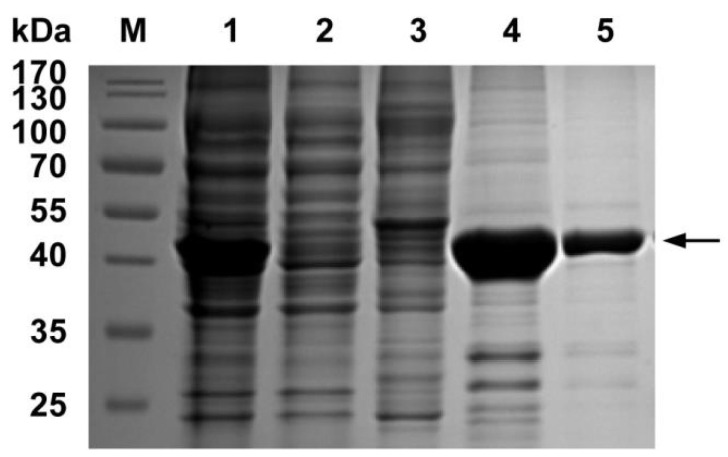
Expression and purification of the PDCoV N protein. Lane M, protein marker; Lane 1, induced *E. coli* BL21 (DE3) with pET-28a-PDCoV-N; Lane 2, induced *E. coli* BL21 (DE3) with empty vector pET-28a; Lane 3, precipitation of the PDCoV N protein strain after ultrasonication; Lane 4, supernatant of the PDCoV N protein strain after ultrasonication; Lane 5, purified PDCoV N protein.
